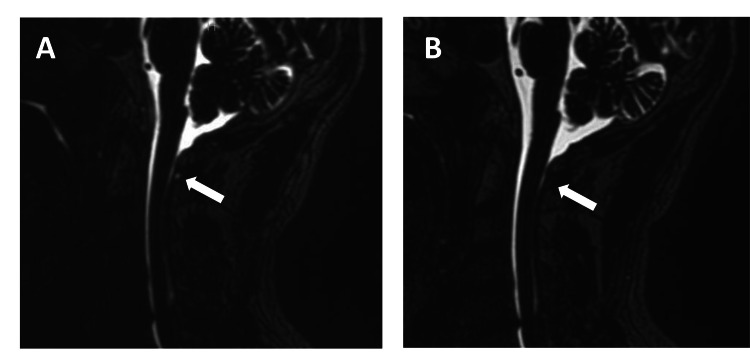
Cervical MRI image. Cervical MRI image of before (A) and after EBP (B). White arrow indicates CSF leak around C1-2 (A). The CSF leakage is not observed after EBP (white arrow) at the same point (B). EBP: epidural blood patch.
